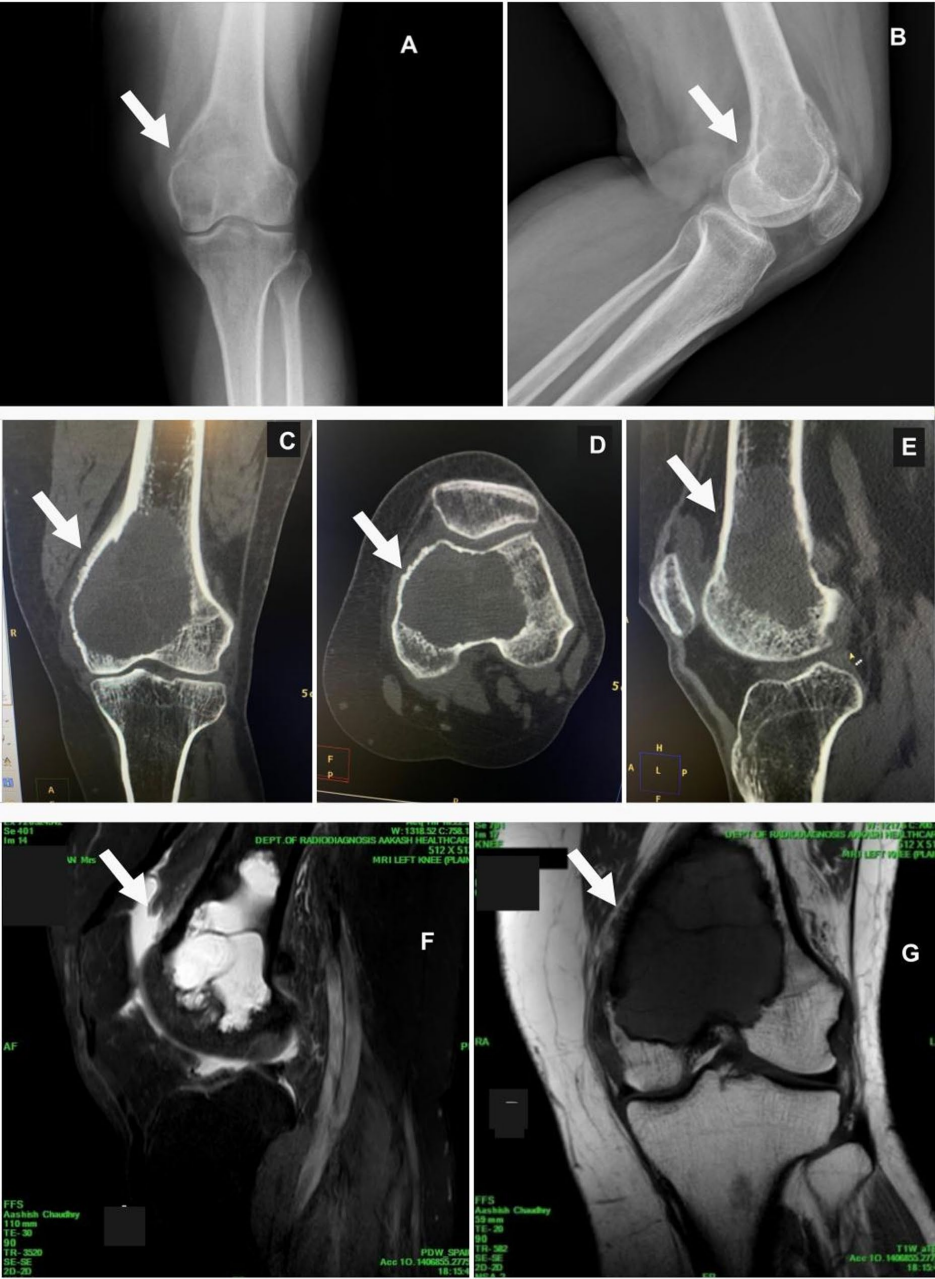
Preoperative radiographic images of the patient with a femoral GCT. (A, B): Show a large, lobulated, lytic, and expansile lesion in the distal femur on X-ray, with a multicystic appearance, internal septations, cortical thinning, and mild periosteal thickening along the medial cortex. The tibiofemoral joint spaces are normal. Differential diagnosis includes giant cell tumor (GCT) or aneurysmal bone cyst (ABC). (C), (D) & (E): reveals a similar lesion with multicystic features, posterior cortical destruction, soft tissue bulging, periosteal thickening, cortical thinning, and knee joint effusion. Tibial structures are normal. These findings also suggest GCT or ABC. (G) & (F): shows a lesion with fluid-fluid levels, internal septations, and hyperintense signals on T2-weighted/STIR images. Femoral cortex thinning, focal destruction, soft tissue bulging, marrow edema, and mild periosteal thickening are noted, with no signs of metastasis or neurovascular invasion, indicating a benign pathology. The findings are consistent with GCT or ABC, requiring careful surgical planning
